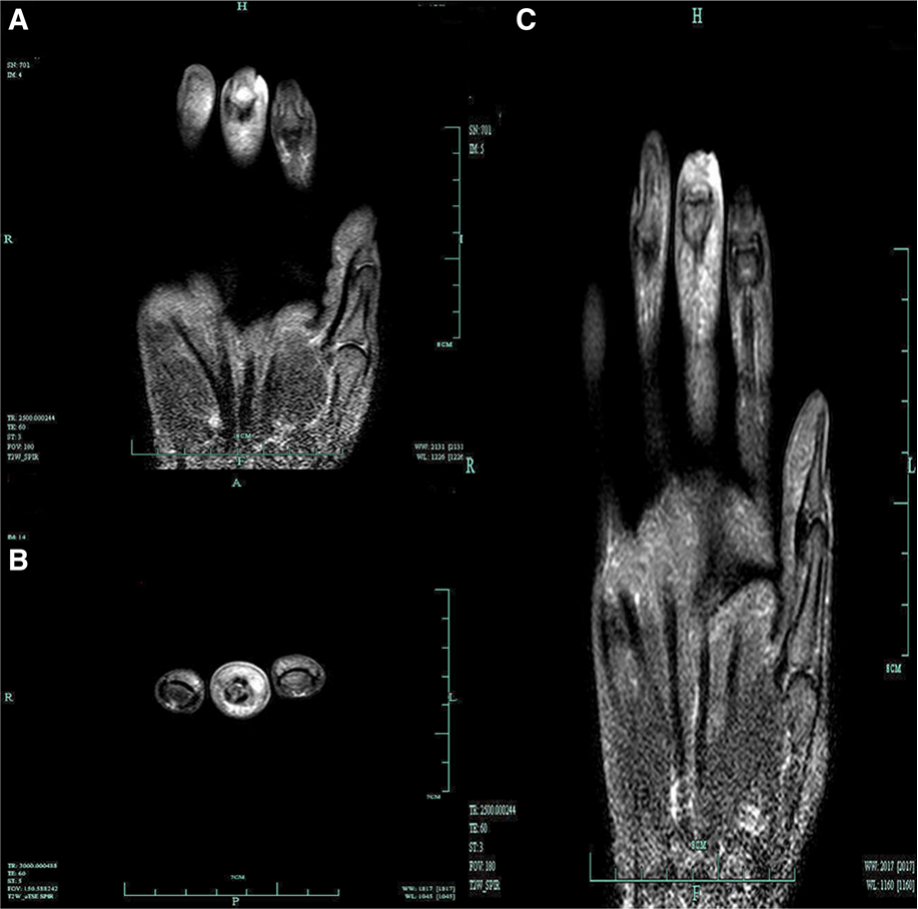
Magnetic resonance imaging (MRI) reveals a subungual approximately solid, well-delimited nodule with hyperintense on the coronal T2-weighted image (a) and the axial T2-weighted image (b). Soft tissue of the distal middle finger is hyperintense on the coronal T2-weighted image (c)
